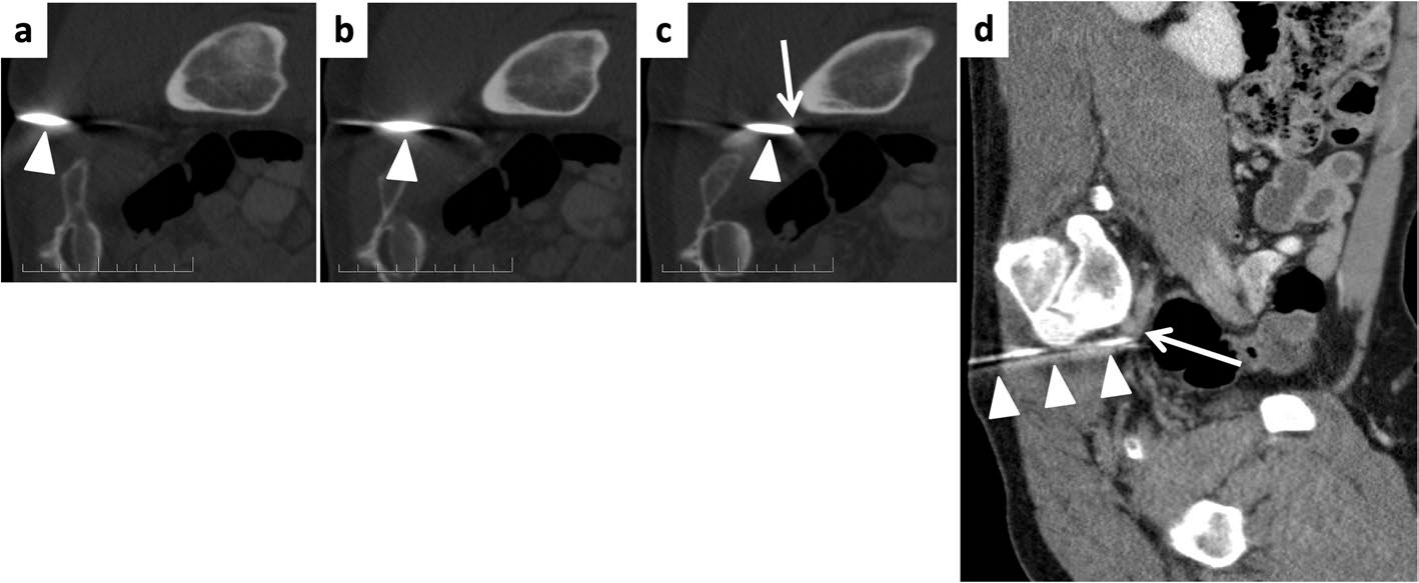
Serial axial (a-c) coronal (d) computed tomography angiography (CTA) with the patient in lateral decubitus position demonstrating the bone marrow biopsy needle (arrowheads) traversing the greater sciatic foramen with its tip within the superior gluteal artery (arrow). Note the absence of surrounding hematoma due to the tamponade effect of the needle
